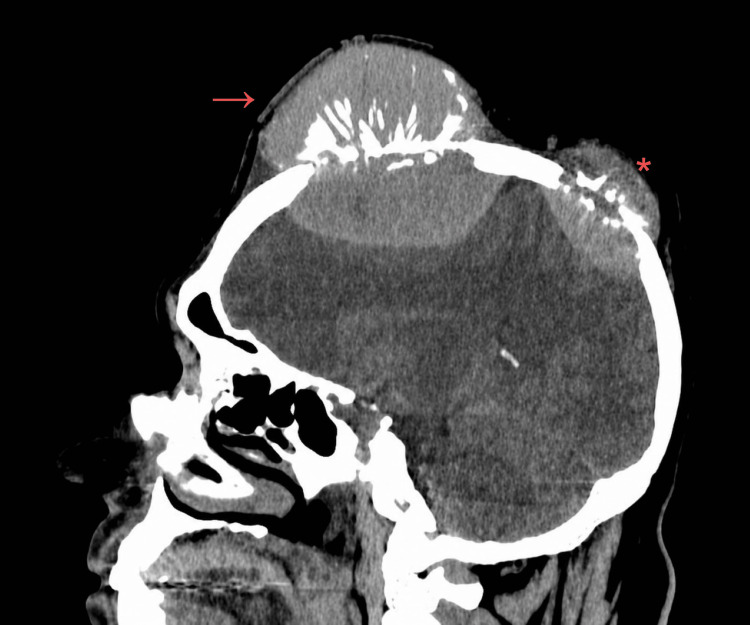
Sagittal CT image demonstrating multicentric calvarial osteolytic lesions Sagittal CT image showing two calvarial osteolytic lesions. The arrow indicates the dominant frontoparietal expansile osteolytic mass, characterized by bone destruction, soft-tissue extension, and internal bony spicules. The asterisk identifies the second smaller lesion in the left posterior parietal region, supporting multicentric calvarial involvement. CT: computed tomography
